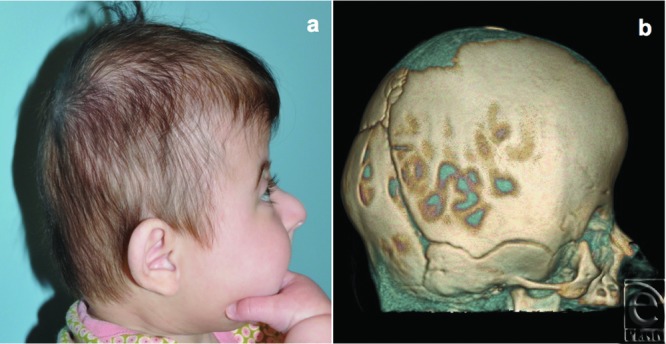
Three-month-old female patient with Saethre-Chotzen syndrome and bicoronal synostosis resulting in significant turribrachycephaly (*a*). The sagittal, lambdoid, and squamosal sutures were patent with a large fontanelle, characteristic of Saethre-Chotzen syndrome. Initial computed tomographic volume rendering shows bicoronal craniosynostosis (*b*).
